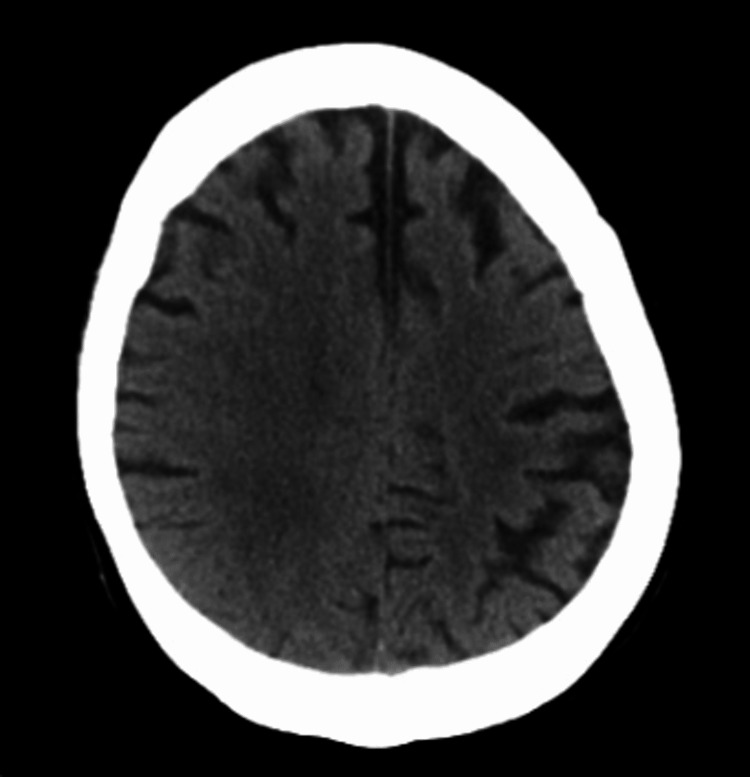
CT scan of the head revealed diffuse parenchymal volume loss. CT: computerized tomography
